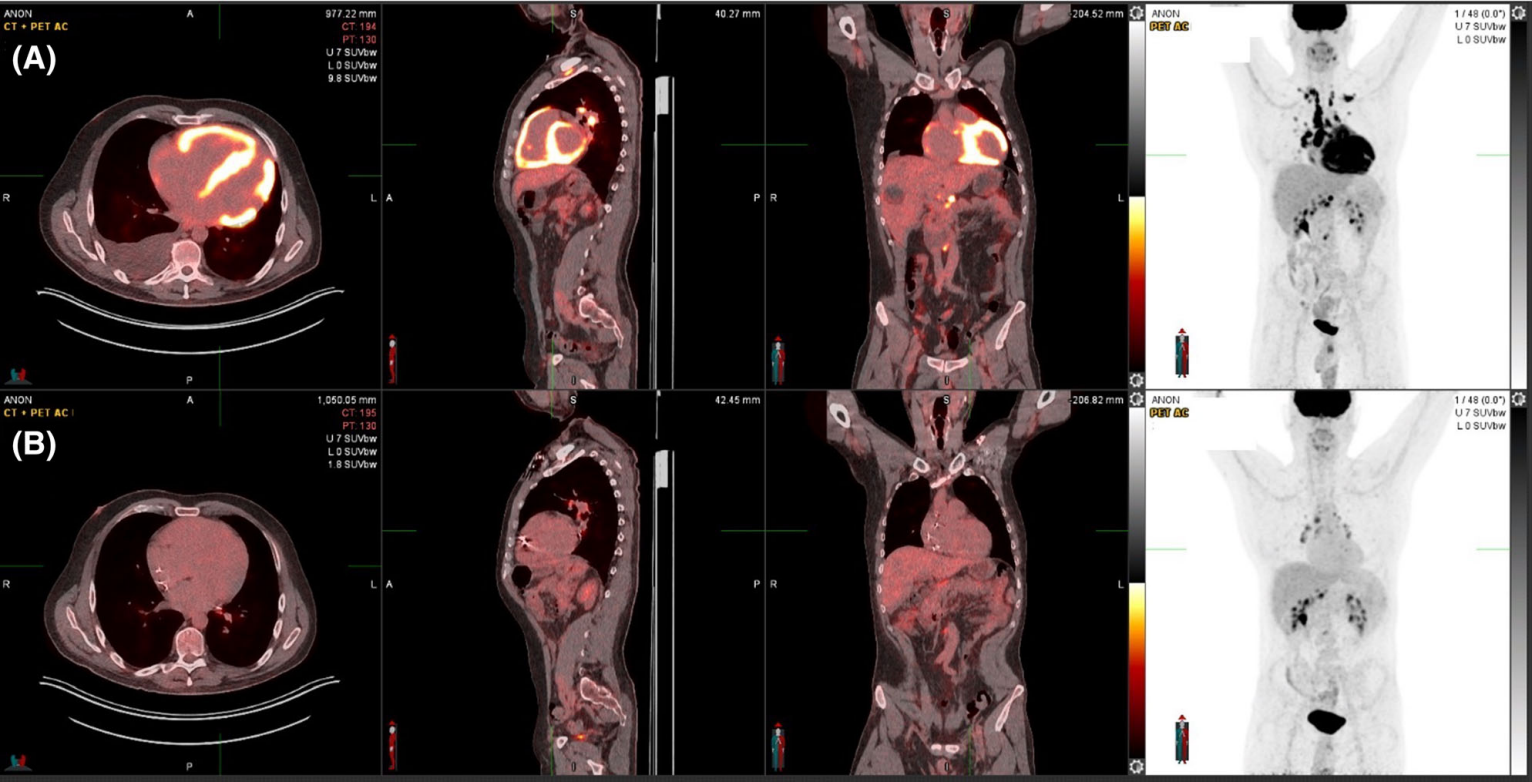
Response to immunosuppressive therapy. FDG-PET at baseline (A) with intense myocardial inflammation sparing only parts of the lateral wall and apex and 6 months after treatment (B). FDG-PET = 18F-fludeoxyglucose positron emission tomography
